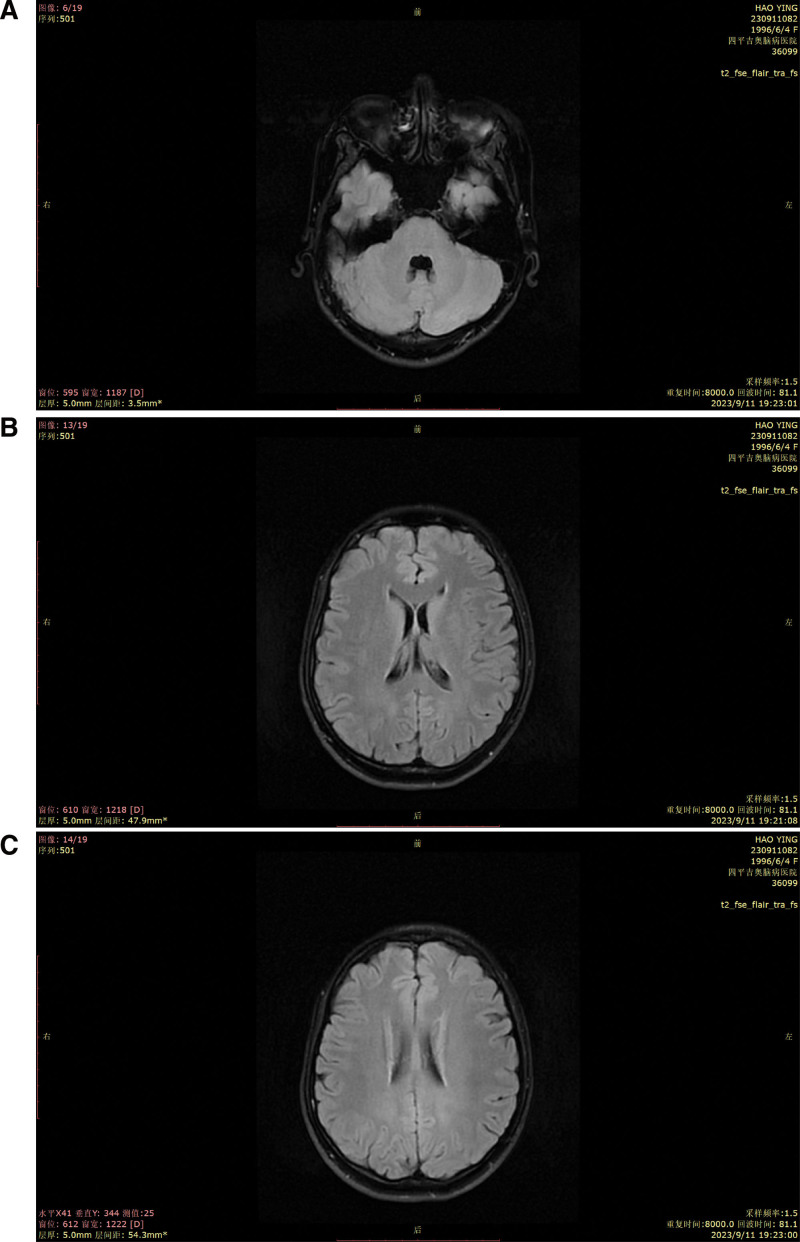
Brain MR exam. T2-weighted MRI showed (A) no abnormalities in the cerebellum and (B and C) white matter lesions around the posterior horn of the lateral ventricle on both sides. MRI = magnetic resonance imaging.
